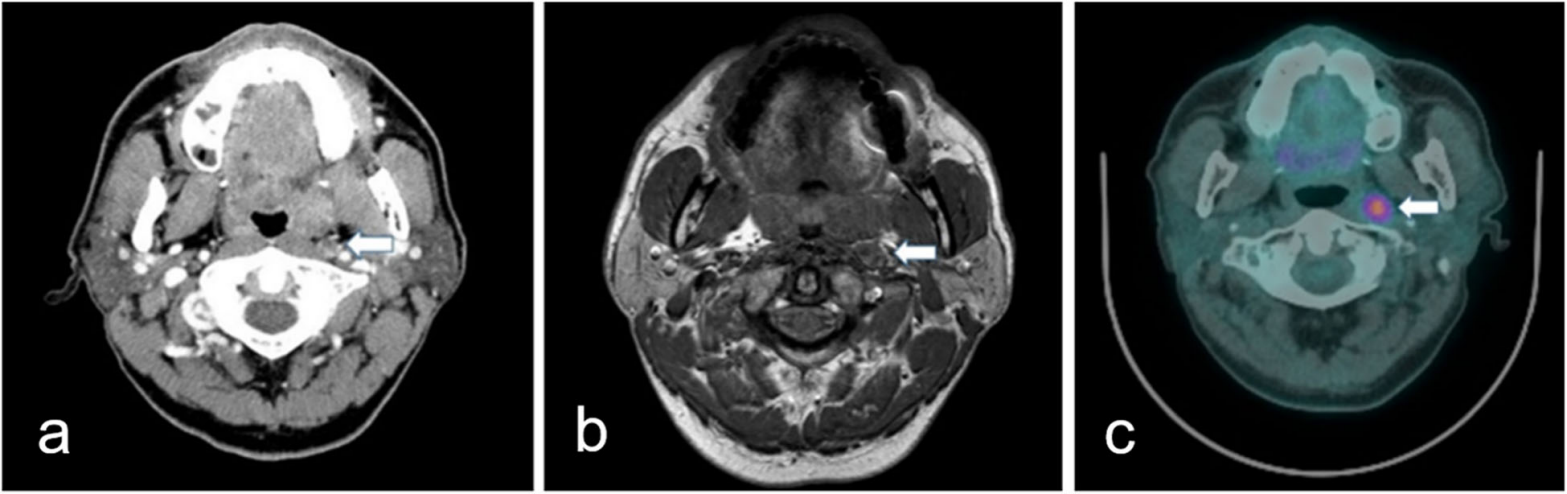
Representative true positive imaging findings of retropharyngeal lymph node. a Contrast-enhanced computed tomography shows a left metastatic retropharyngeal lymph node (*arrow*). b T1-weighted gadolinium-enhanced magnetic resonance imaging shows a left metastatic retropharyngeal lymph node (*arrow*). c Positron emission tomography-computed tomography shows an abnormal ^18^fluorine-fluorodeoxyglucose uptake with a standardized uptake value of 3.1, on a left retropharyngeal lymph node (*arrow*)
